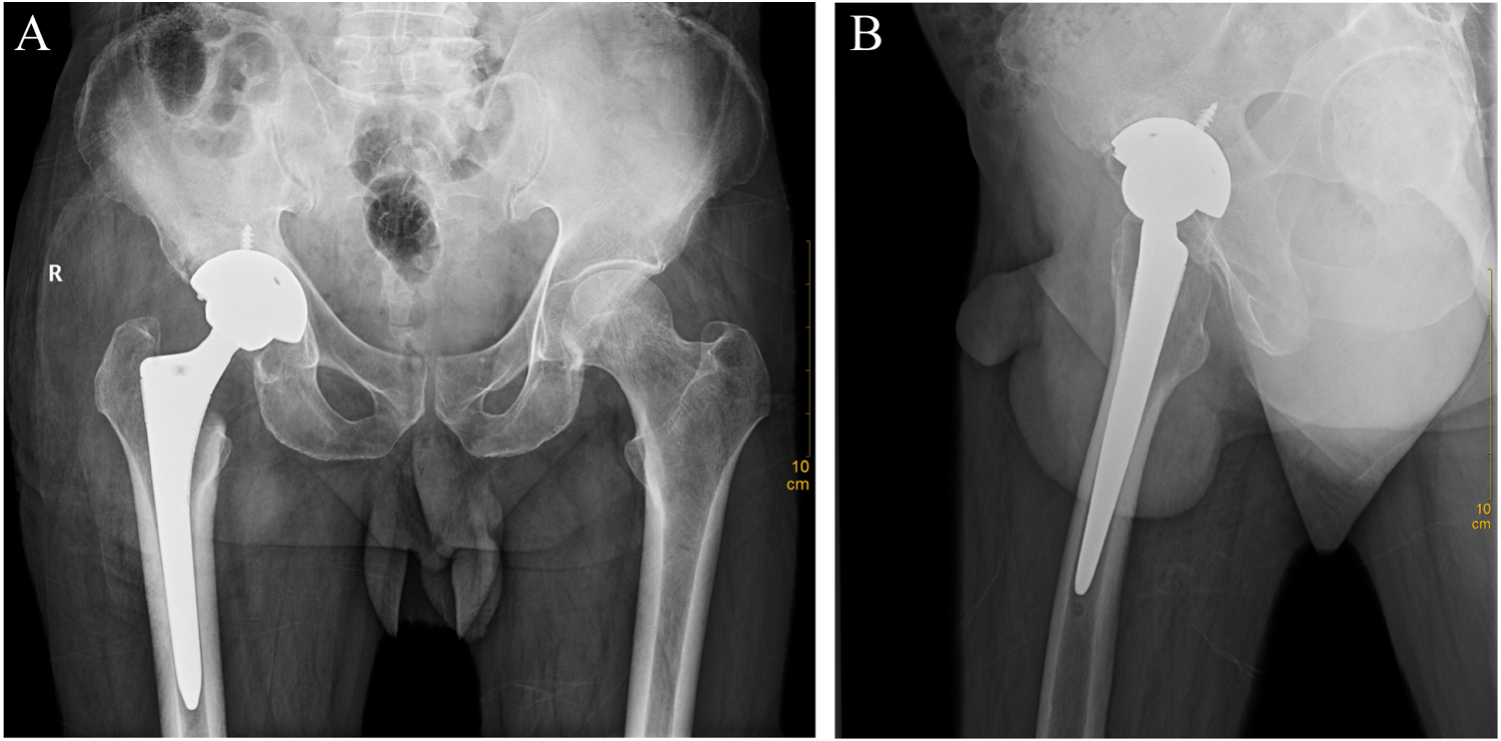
Anteroposterior (A) and lateral (B) radiographs of the hip joint 3 years after total hip arthroplasty surgery.
